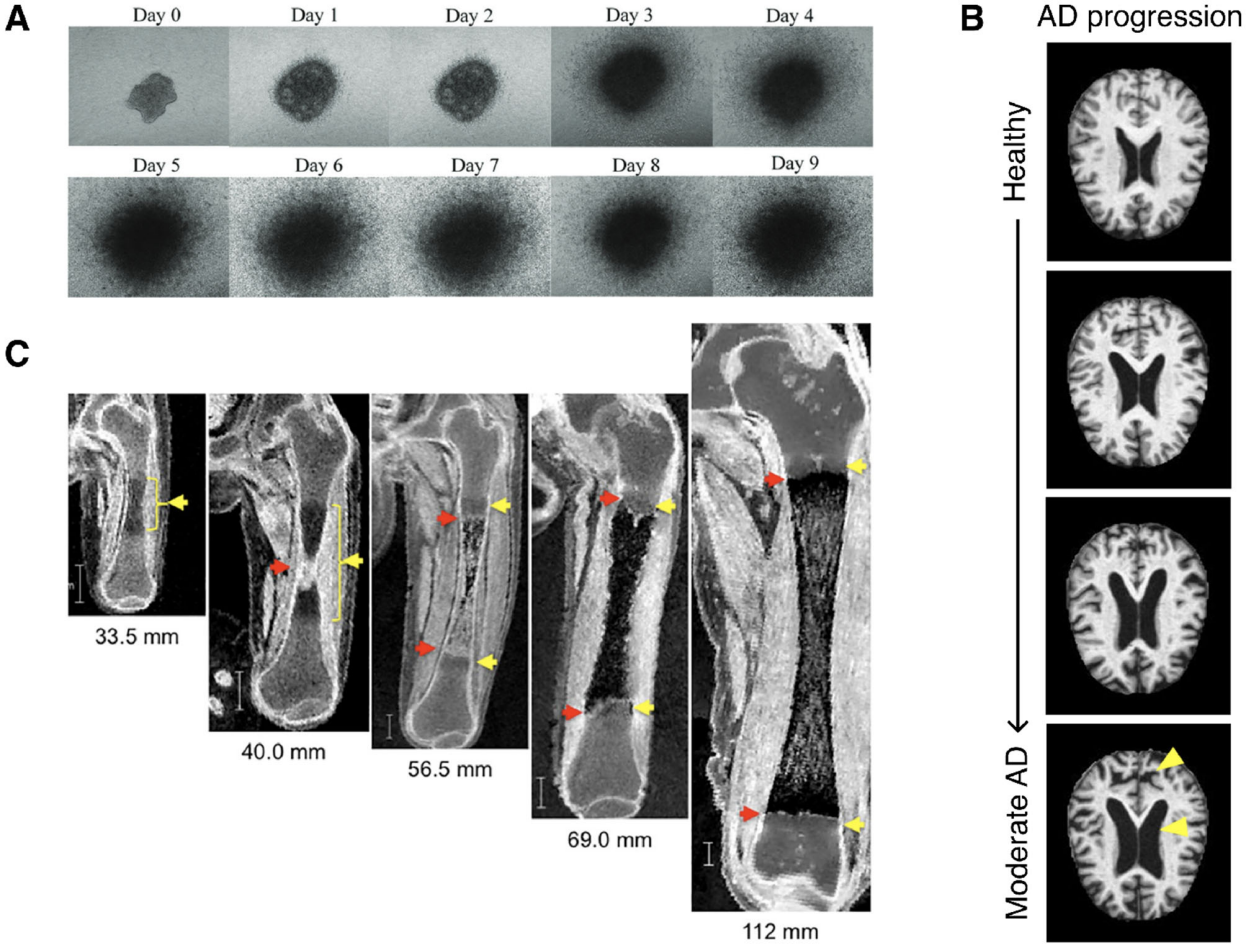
(A) Longitudinal phase contrast imaging of 3D cell cultured cervical cancer spheroids (Muniandy et al., 2021)*. (B) Neurodegradation of brain structure with progression of AD, from healthy to moderate AD (top to bottom). Adapted from Pasnoori et al. (2024)*. (C) Longitudinal MRI imaging of the morphogenesis of a femur during the embryonic and fetal periods. Figure adapted from Suzuki et al. (2019)*. *Images obtained from referenced sources and licensed under CC BY 4.0 (https://creativecommons.org/licenses/by/4.0/).
